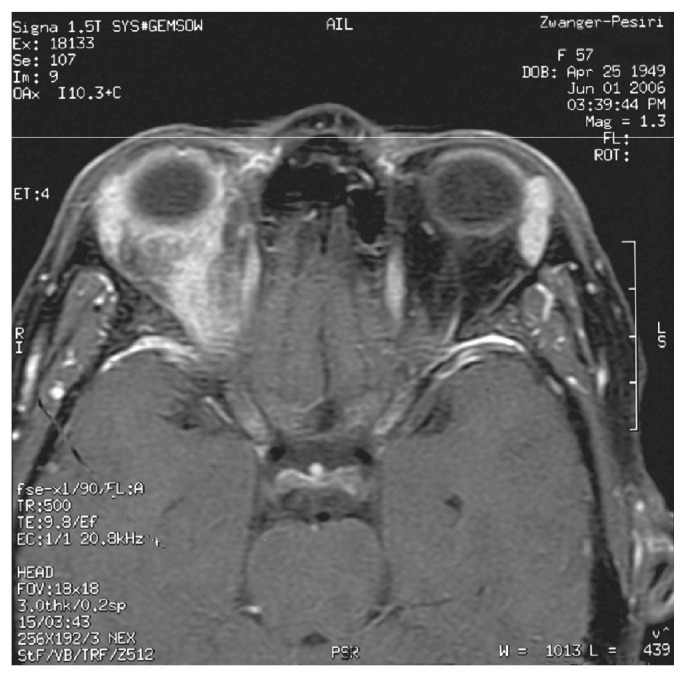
MRI of brain and orbit which reveals enhancement of soft tissue intimately associated with right optic nerve sheath.
